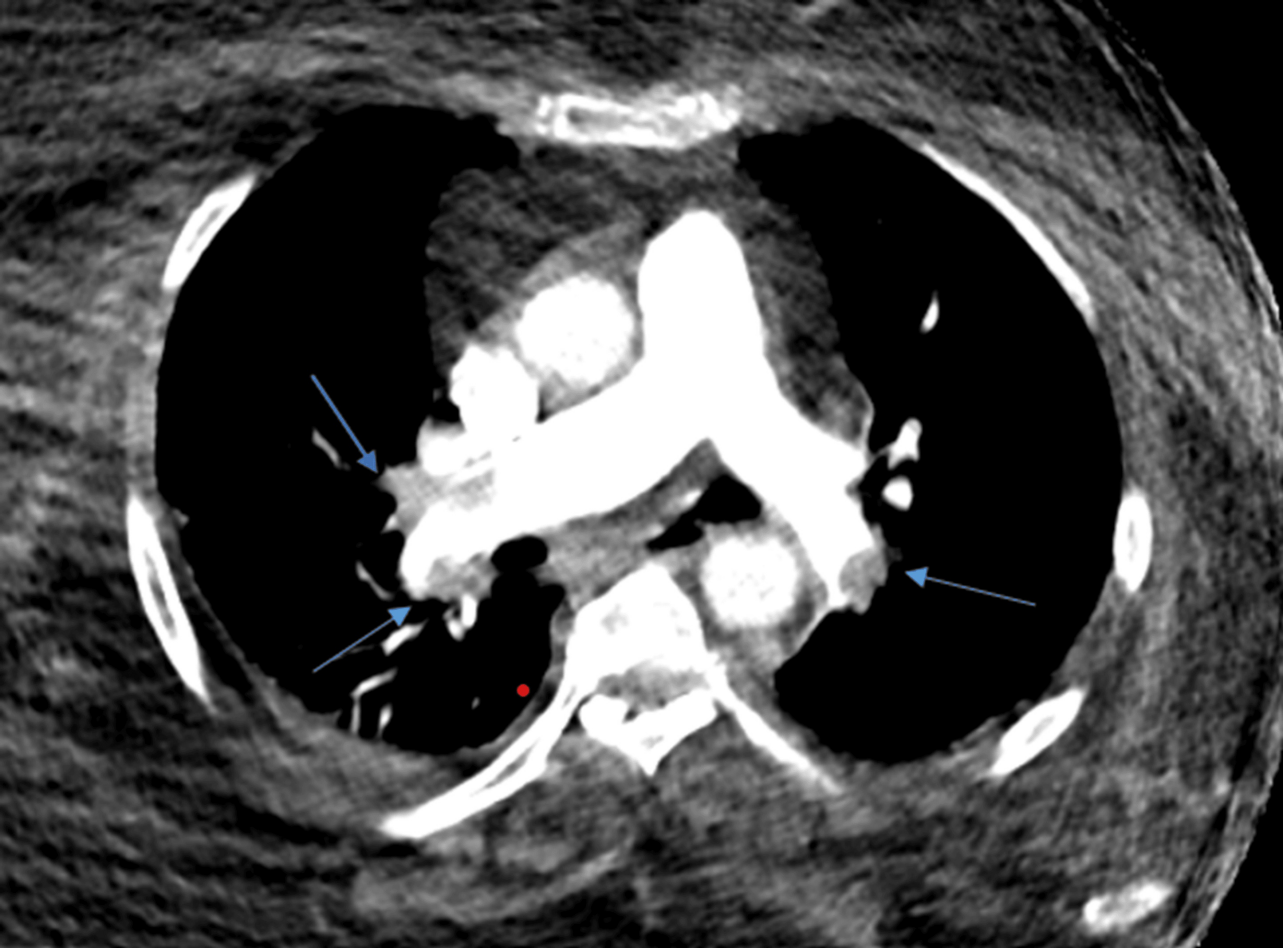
CTPA confirming bilateral acute pulmonary embolism (blue arrows) CTPA: computed tomography pulmonary angiography
